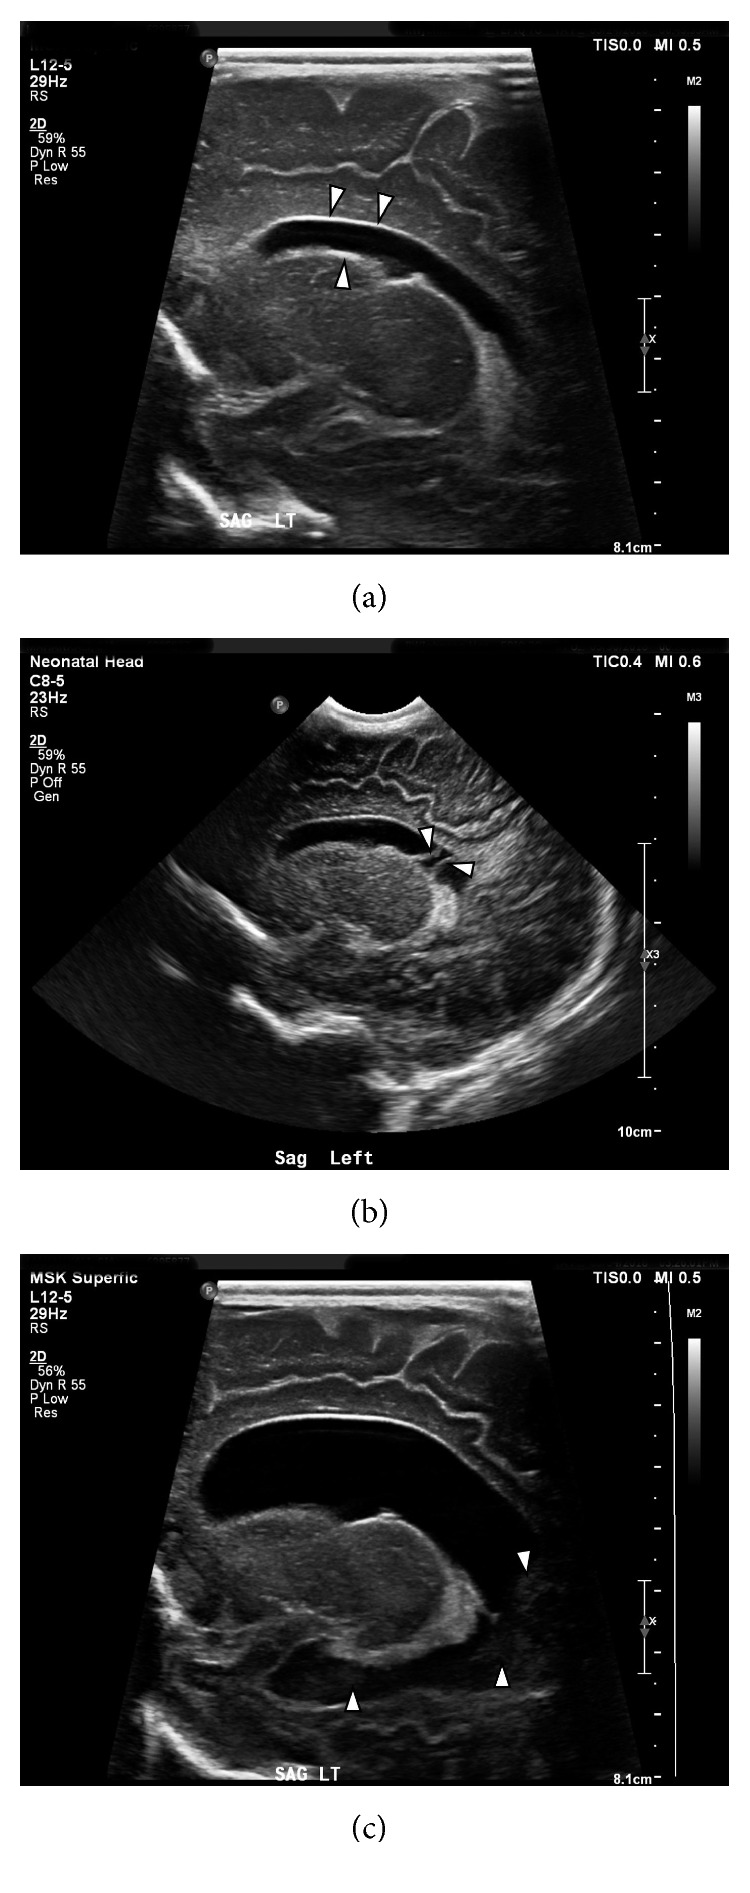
(a) Neurosonogram performed on the 2nd hospital day showed a mildly dilated left lateral ventricle and echogenic ependyma (arrows); findings associated with ventriculitis. (b) Hospital day 7: neurosonography showing mildly dilated left lateral ventricle, echogenic ependyma, and intraventricular debris (arrows), suggestive findings associated with ventriculitis. (c) Hospital day 11: neurosonography showing moderately dilated left lateral ventricle, echogenic ependyma, and intraventricular debris (arrows).
